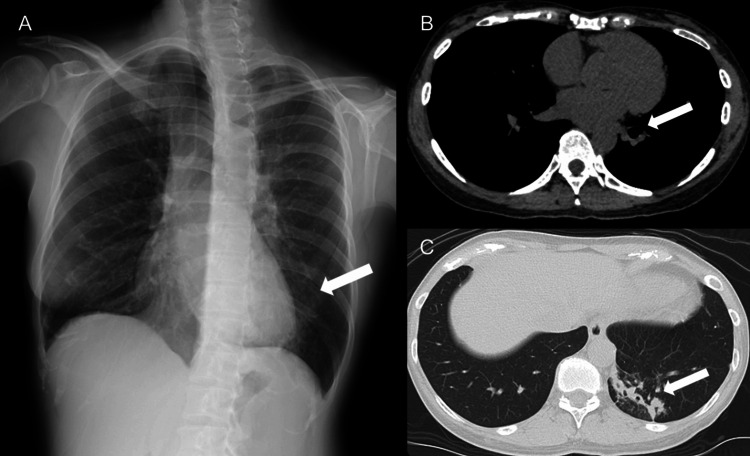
Imaging findings three weeks after bronchoscopy (A) Chest plain radiograph, second oblique view, three weeks after bronchoscopy. Improvement in the transparency of the left lower lung field is observed (arrow). (B, C) Chest plain computed tomography scan three weeks after bronchoscopy. No residual foreign body is detected after bronchoscopy, and the infiltrative opacities show a trend toward improvement (arrow).
